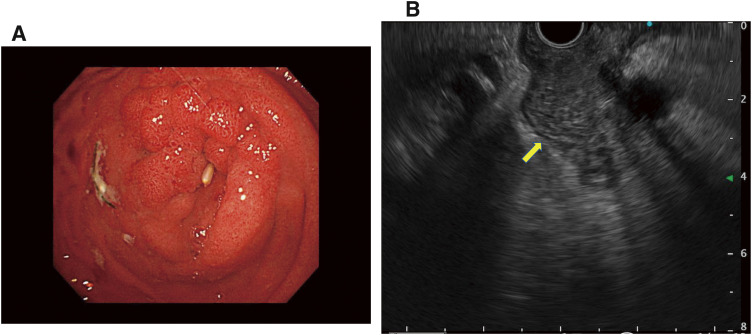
Esophagogastroduodenoscopy and endoscopic ultrasonography findings. (A) Esophagogastroduodenoscopy showed duodenal obstruction at the first visit. (B) Endoscopic ultrasonography showed wall thickening in the second portion of the duodenum. The yellow arrow showed the thickening duodenal wall.
